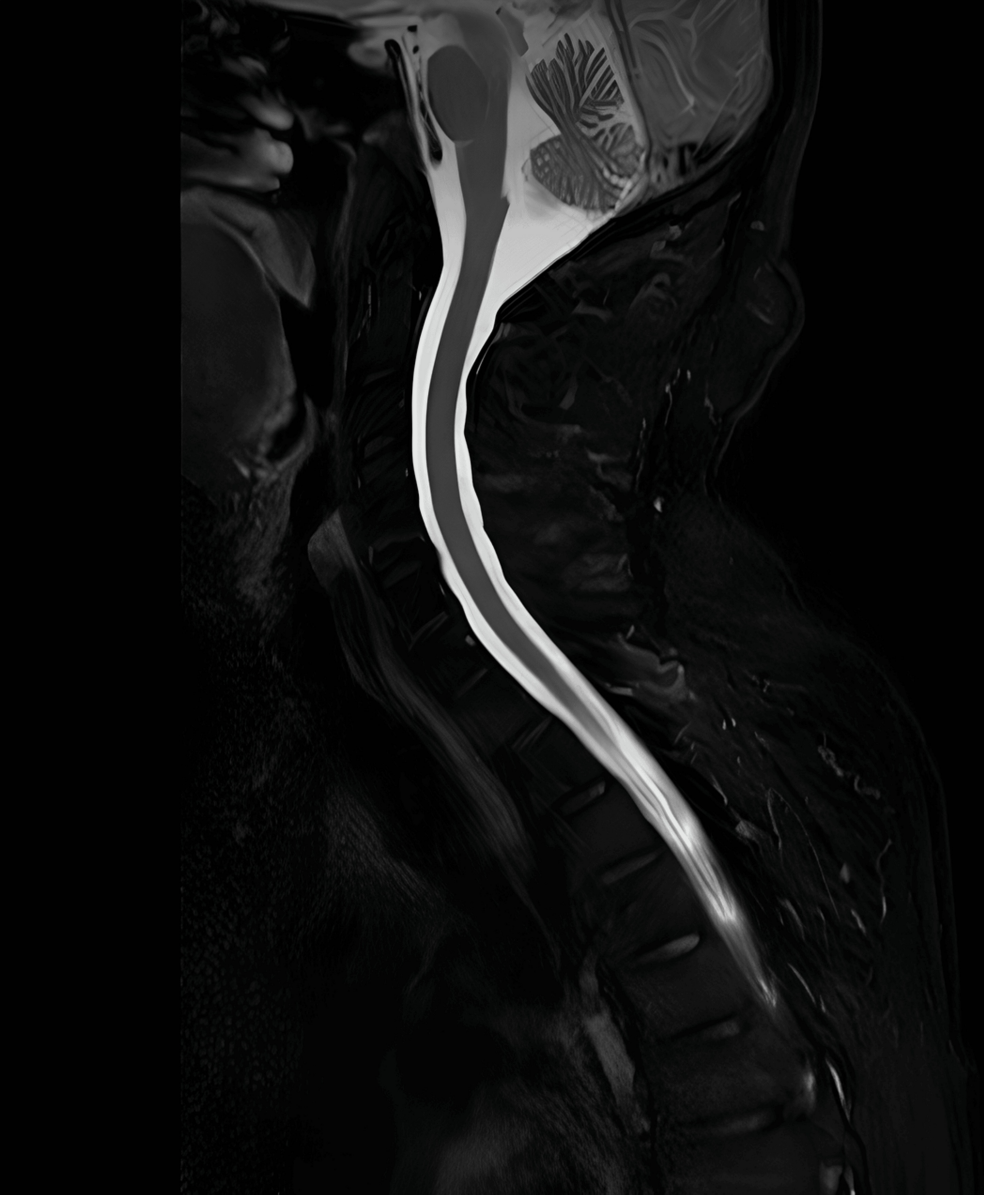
MRI of the entire spine (T2-weighted fluid-attenuated inversion recovery sequence; upper section shown), demonstrating preserved vertebral body height, alignment, and marrow signal, with no evidence of disc pathology, spinal canal stenosis, cord compression, or abnormal enhancement.
